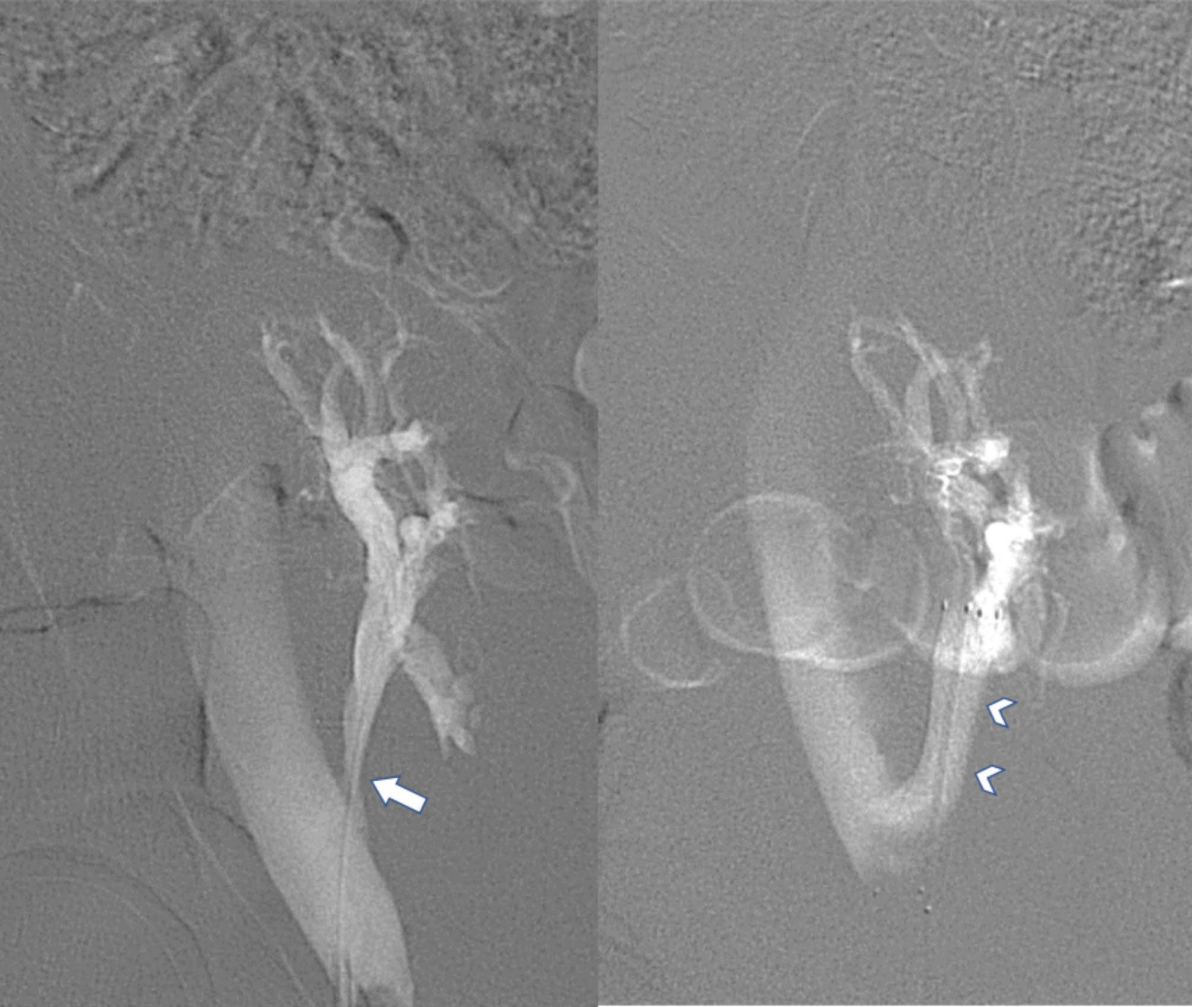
a: DSA Graft vein stenosis corresponds with CTA results [arrow]. b: Self expandable stent placed to the graft vein [arrowheads]
